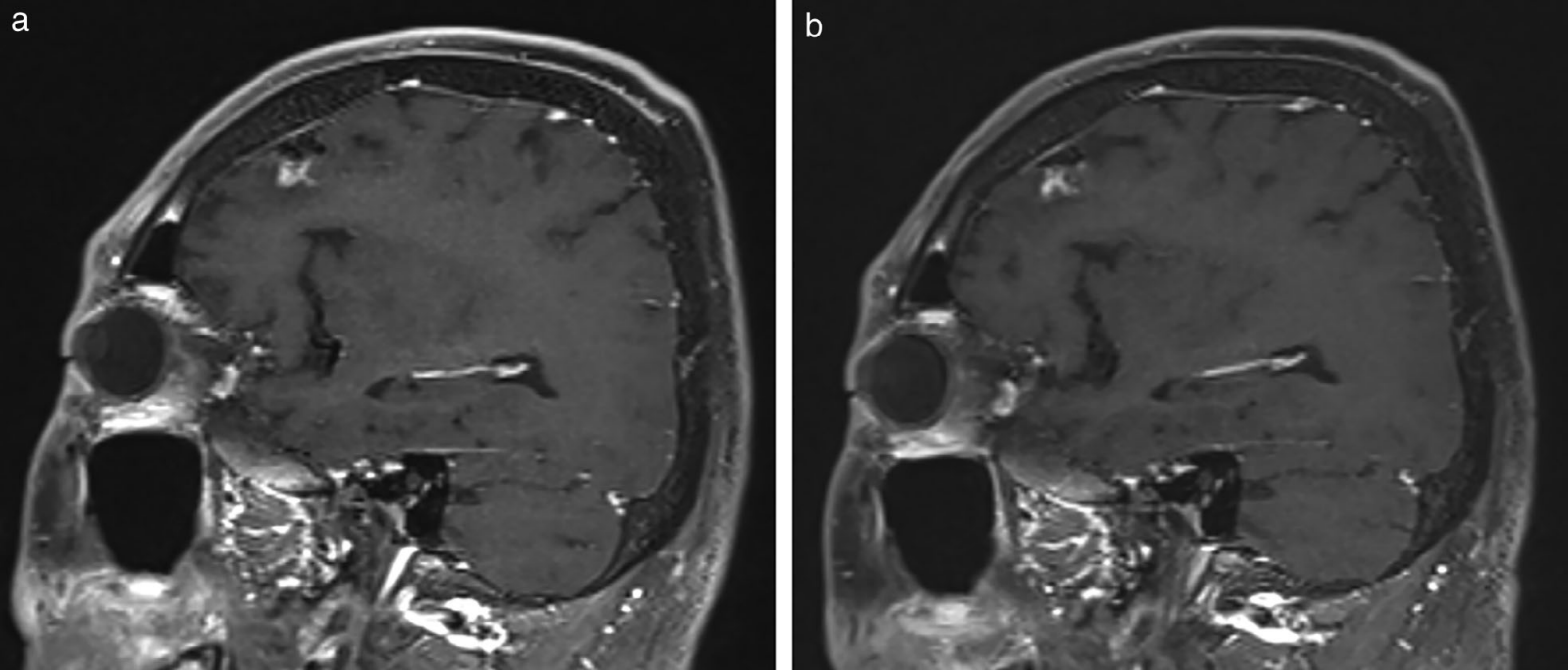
Brain enhanced magnetic resonance imaging (a) before; and (b) after four cycles of chemoimmunotherapy induction with atezolizumab, carboplatin, and etoposide. The shrinkage of brain metastasis after four induction therapies was limited, with a stable disease.
